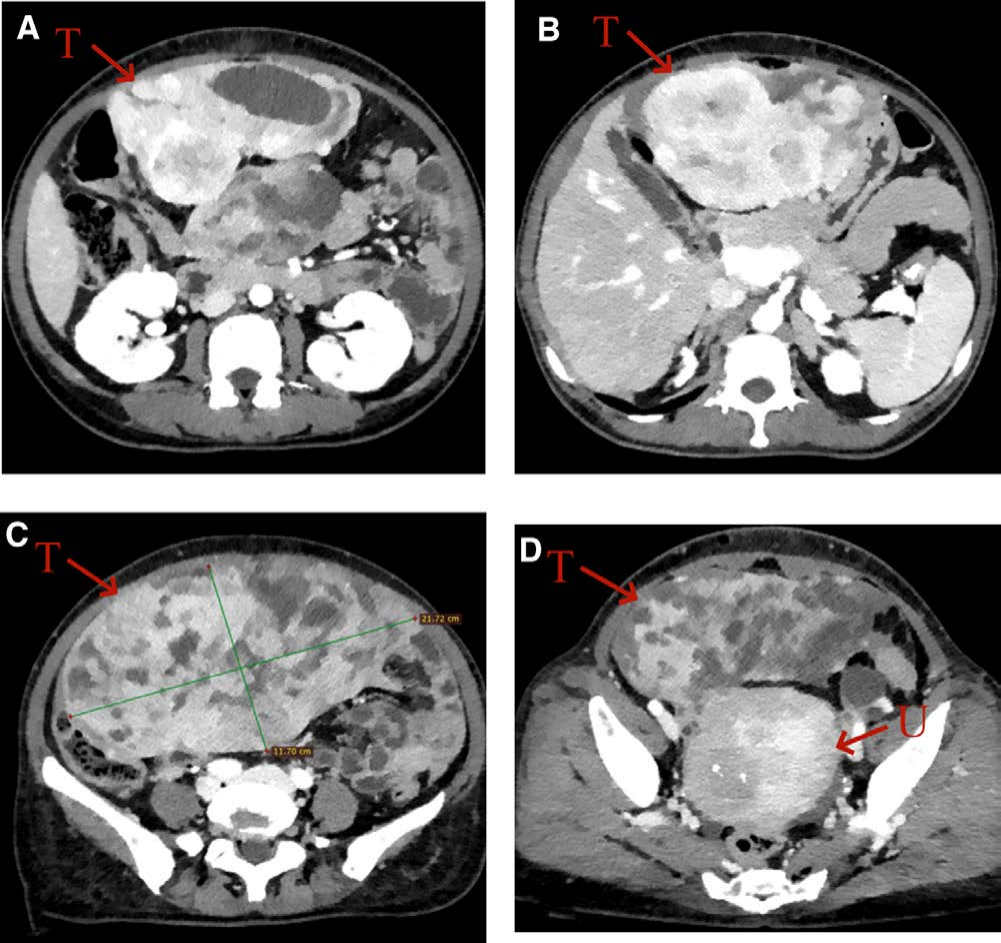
Enhanced CT of the abdomen. (A) and (C) Preoperative enhanced CT: the axial plane of the abdominal pelvic tumor. (B) Postoperative CT: no evidence of recurrence and metastasis. T = tumor, U = uterus.
